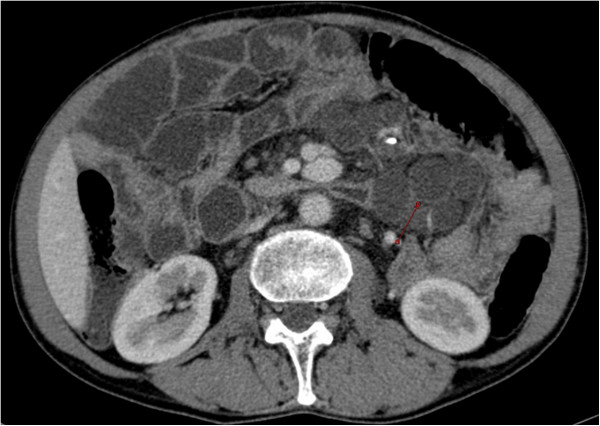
Computed tomography – cystic mesenteric masses - central low attenuation with enhanced rims (arrow).
